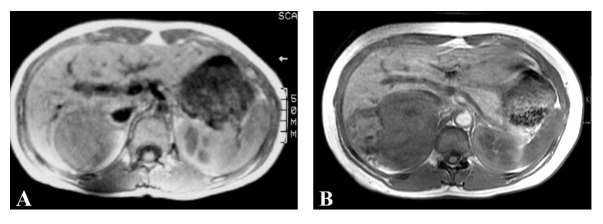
Serial MRI examinations spanning 18 years, showing low signal mass in the right adrenal gland which is irregular and heterogeneous on (A) Axial T1 spin echo measuring 6 cm in 1992 and (B) Axial T1 inphase gradient echo sequence measuring 14 cm in 2010.
